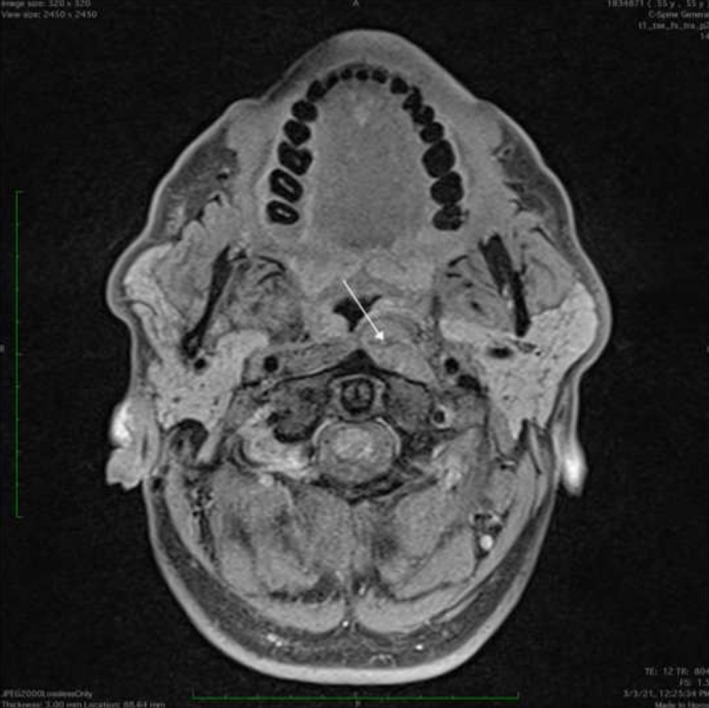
Axial MRI T1 sequence suggestive of a mass in posterior pharyngeal space. (arrow)
